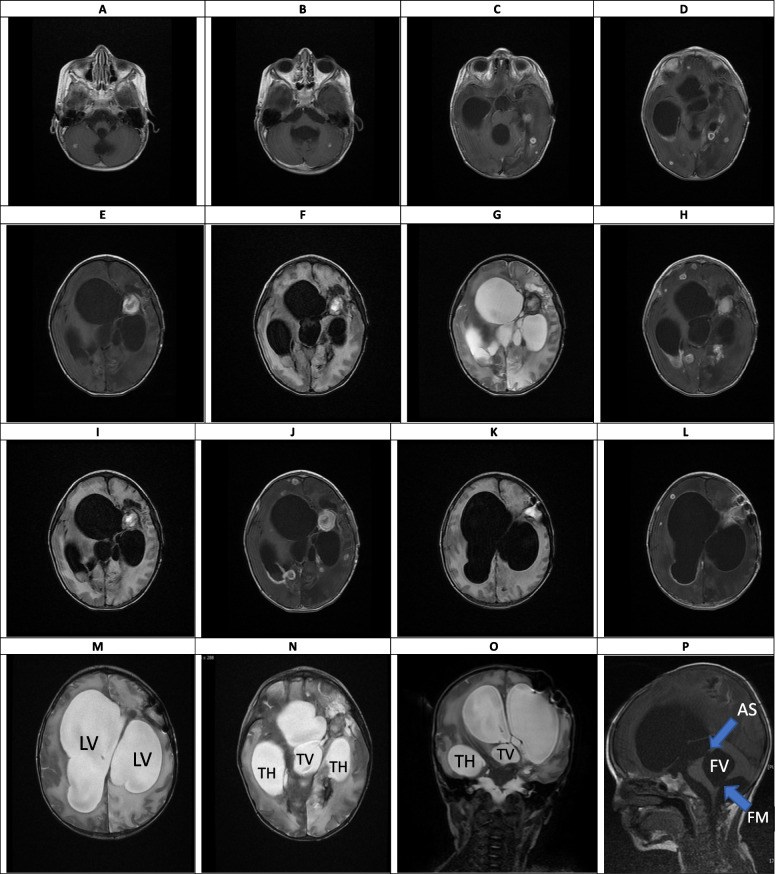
(the Brain MRI Without and with contrast, day 73). A-P Sign of multiple ring-like enhancing lesion is seen at the different parts of the brain parenchyma including cerebellar hemispheres associated with surrounding edema formation suggestive of multiple abscess formation (A-H). Marked enhancement of ependymal layer of the posterior aspect of the right lateral ventricle (at the level of occipital horn and trigone) and also with a lesser degree at left lateral ventricle is seen which are suggestive of diffuse ventriculitis. Some acute hemorrhage is seen at the site of mentioned largest lesion of the left frontotemporal lobe (I-L). Despite of presence of EVD within the right lateral ventricle (not shown here), severe communicating type of hydrocephalus associated with a midline shift to the left side is seen, again suggestive of EVD malfunction or severity of hydrocephalus. Sign of severe dilatation of lateral ventricles (including temporal horns) as well as third and fourth ventricles and also aqueduct of *Sylvius* and foramen *Magendie*, all suggestive of communicating type of hydrocephalus (M-P). LV: lateral ventricle, TH: temporal horn of lateral ventricle, TV: third ventricle, FV: fourth ventricle, AS: aqueduct of *Sylvius*, FM: foramen *Magendie*
